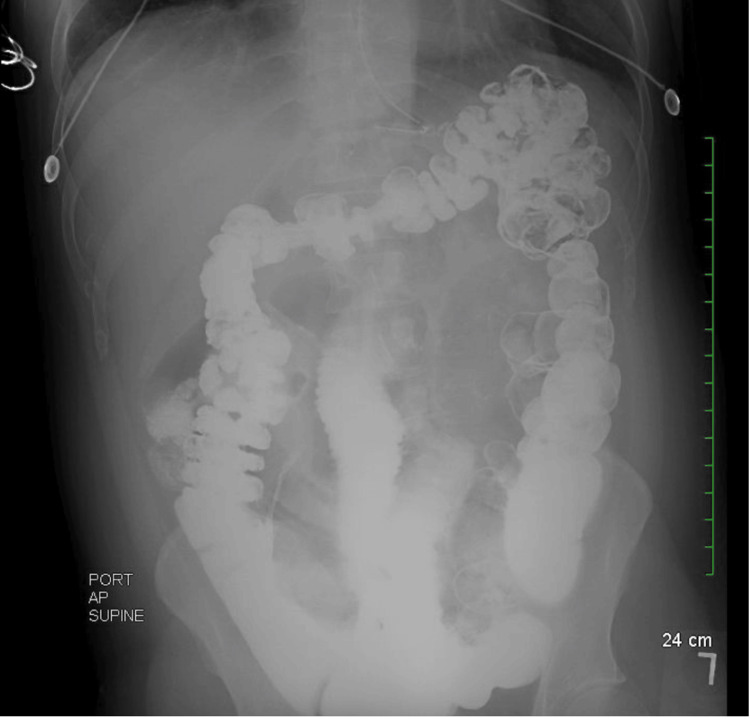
Abdominal X-Ray 24 Hours Post Small-Bowel Follow-Through. An Abdominal x-ray was obtained for comparison with the small bowel follow-through from the previous day. Contrast now projects over the colon. A few distended small bowel loops, which have contrast intraluminally, are still visualized, measuring up to 5 cm in diameter. The NG tube is visualized within the stomach lumen.
